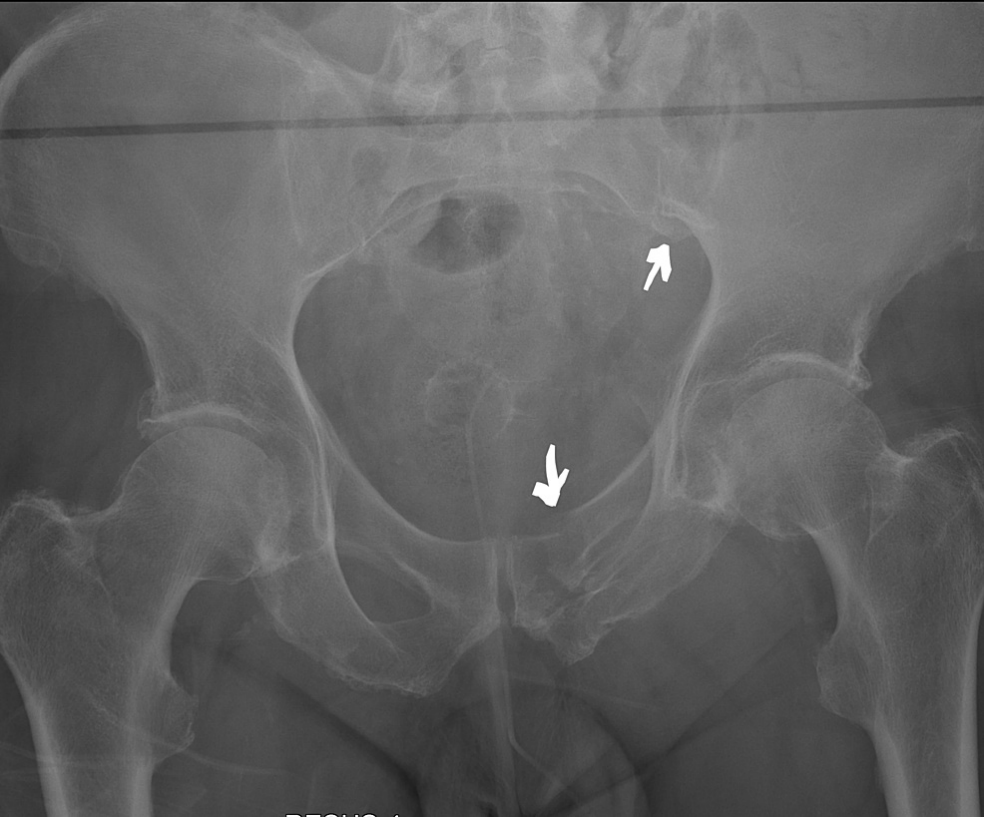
AP Radiograph demonstrating Pelvic Ring injury with Subtle Superior displacement of Left Hemipelvis* (Vertical Shear Fracture) *Arrows indicating points of Fracture and superior displacement of Left Hemipelvis
